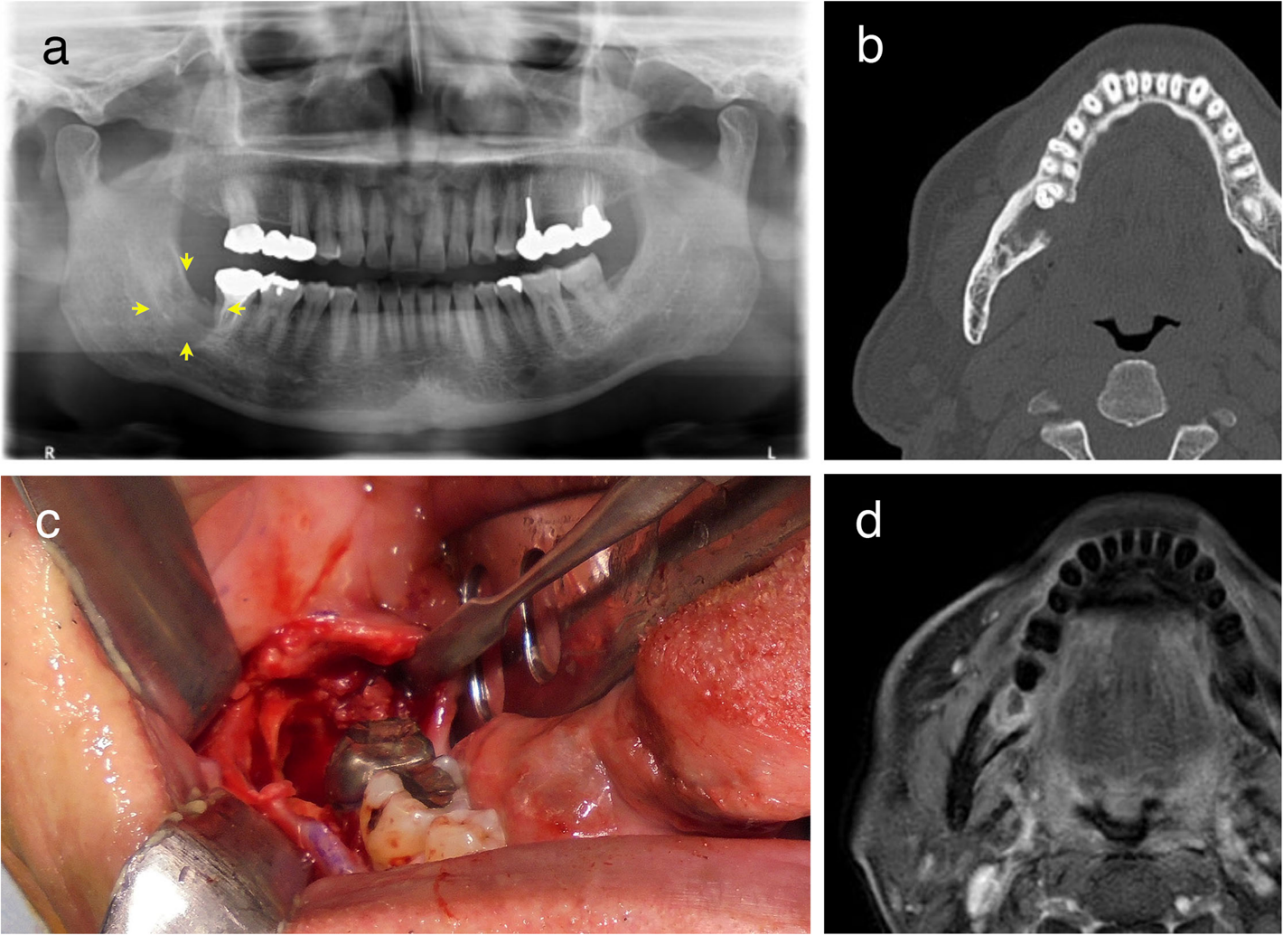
Radiological findings of the central mucoepidermoid carcinoma (MEC) arising from a glandular odontogenic cyst (GOC) of the mandible. a Panoramic radiograph showing a radiolucent cystic lesion (yellow arrows) in the right third molar region of the mandible. b CT showing a unilocular radiolucent lesion with a lingual side cortical bone resorption of the mandible. c Oral photography showing a cystic cavity in the same area after surgery. d MRI showing a contrast defect in the same area after the biopsy was taken
